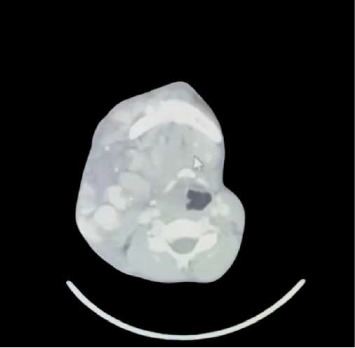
CT scan images revealing the extent of the vascular malformation. The malformation extends from the infratemporal fossa to the thoracic inlet, partially obstructing the oropharynx and nasopharynx, displacing the larynx to the left, and narrowing it slightly.
